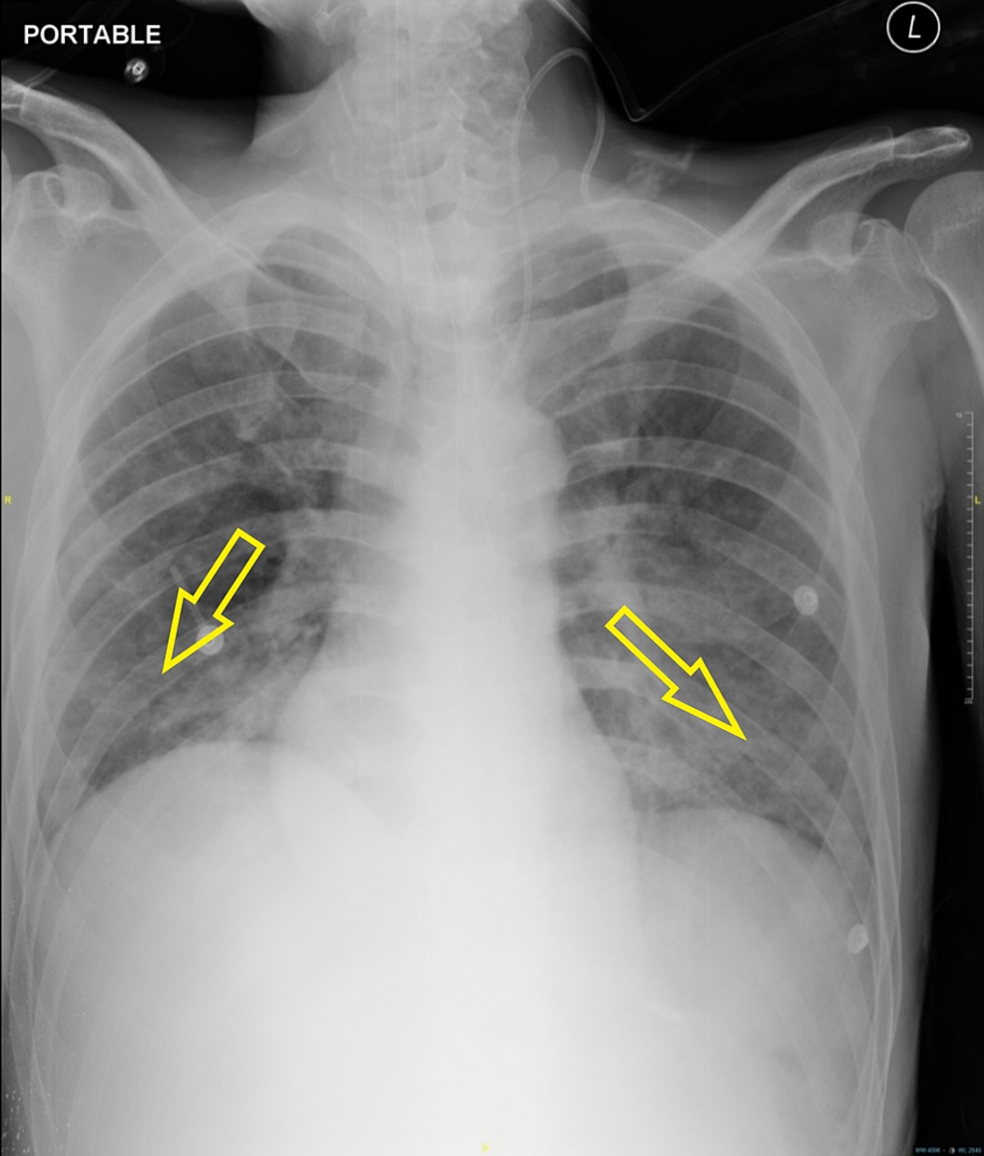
Chest X-ray shows bilateral lower zone ground-glass opacities (yellow arrows).
